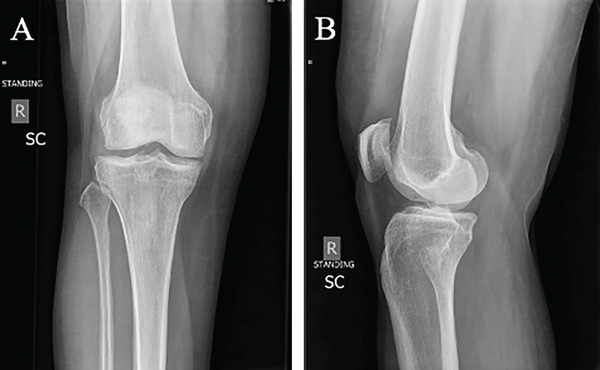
Preoperative radiograph of the right knee showing the osteochondral defect at the lateral femoral condyle from the frontal view (A) and more predominantly from the lateral view (B).
